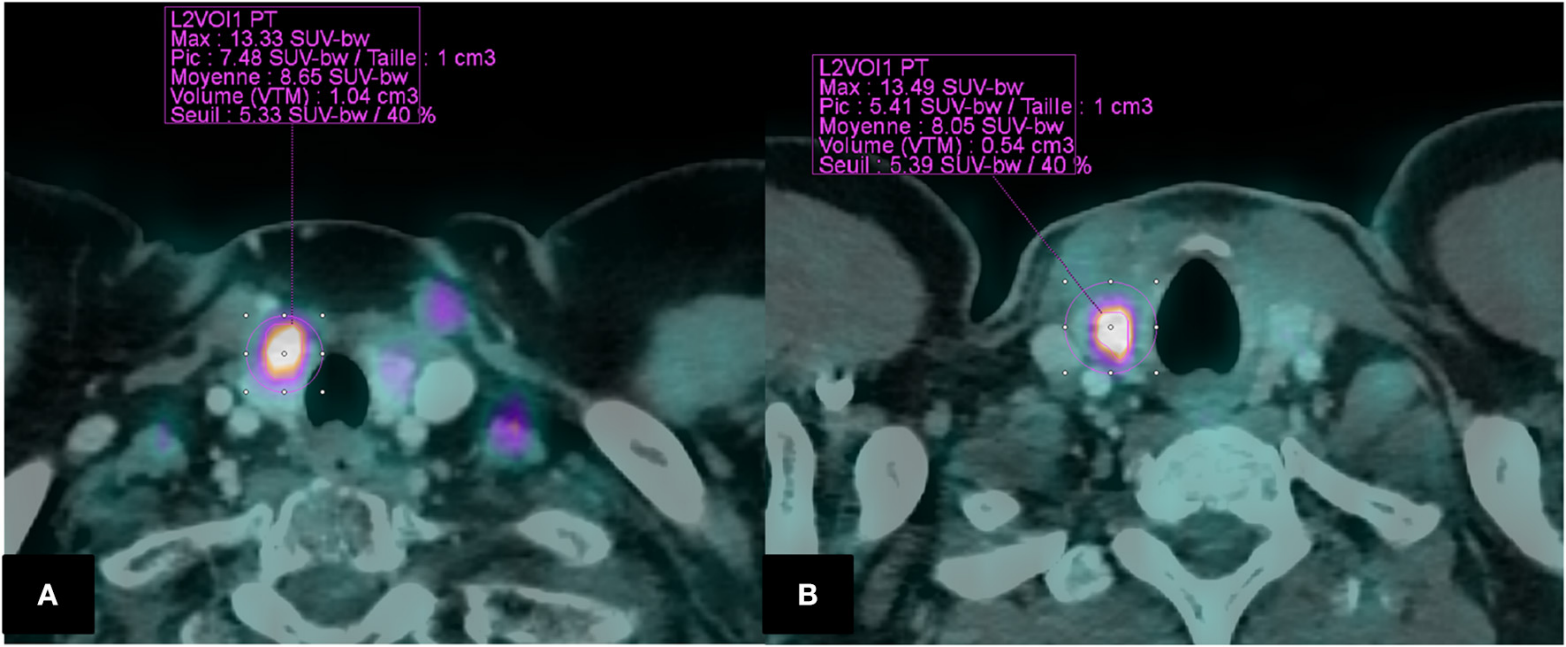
Segmentation of fTI using a fixed SUV threshold method (40 % of SUVmax) Example of two patients (A): BL in a 54,F (SUVmax = 13.33; MTV = 1.04; TLG = 8.99; TBRmax = 7.15; TBRmean = 4.60; TLRmax = 4.22; TLRmean = 2.72; Deauville “like” Scale = 5). (B): ML in a 38,H (SUVmax = 13.49; MTV = 0.54; TLG = 4.34. TBRmax = 7.45; TBRmean = 4.44 TLRmax = 5.81; TLRmean = 3.46; Deauville “like” Scale = 5).
